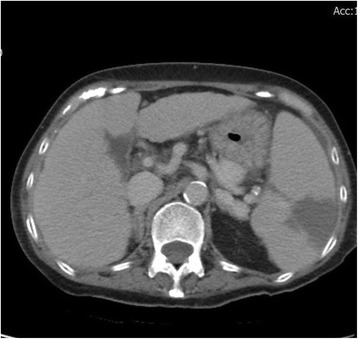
Computed tomography of the abdomen, revealing a splenic infarction
